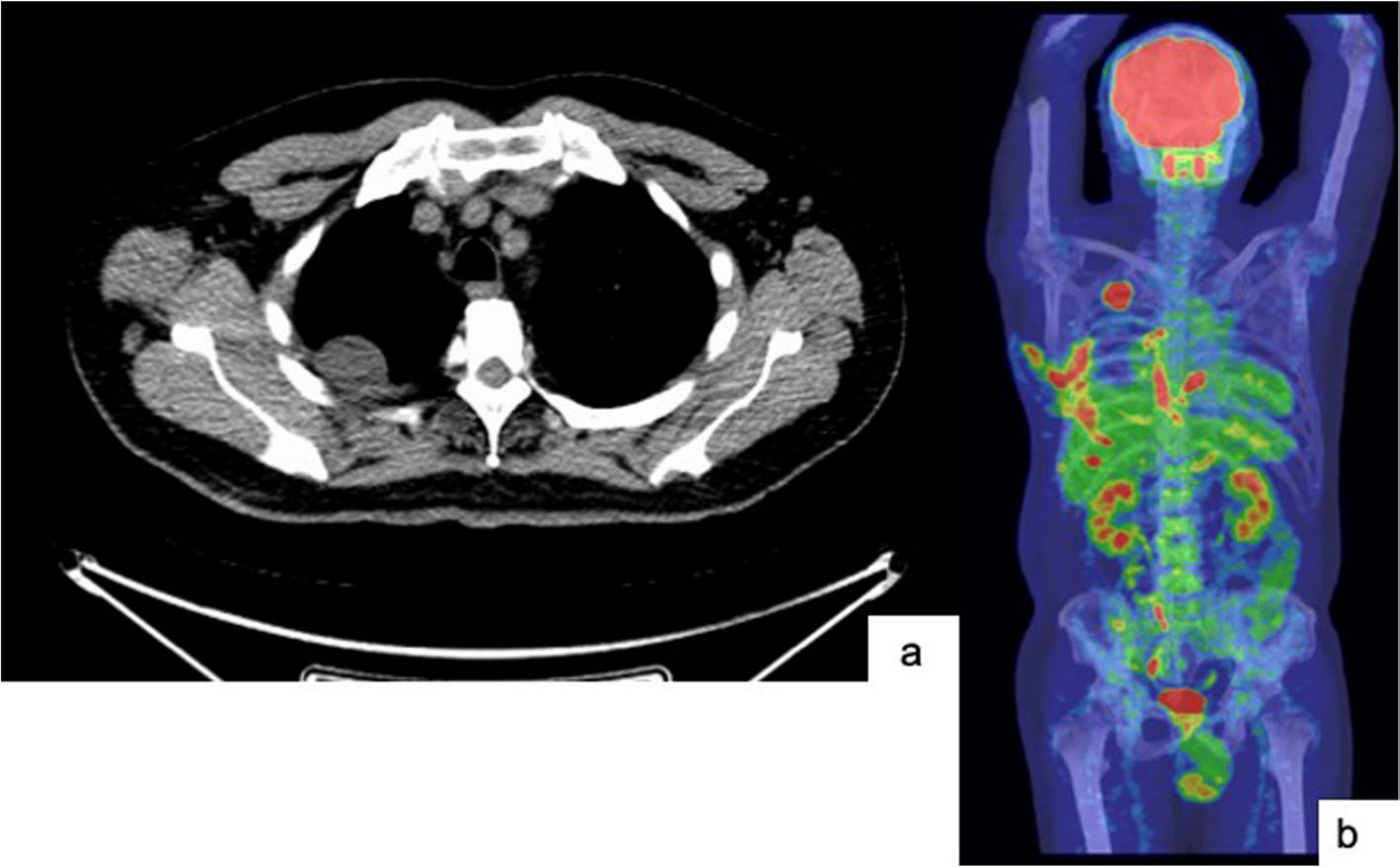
PET-CT at 1 month postoperatively shows considerable pleural dissemination in the right lung field. There was no abnormal uptake in the extremities
